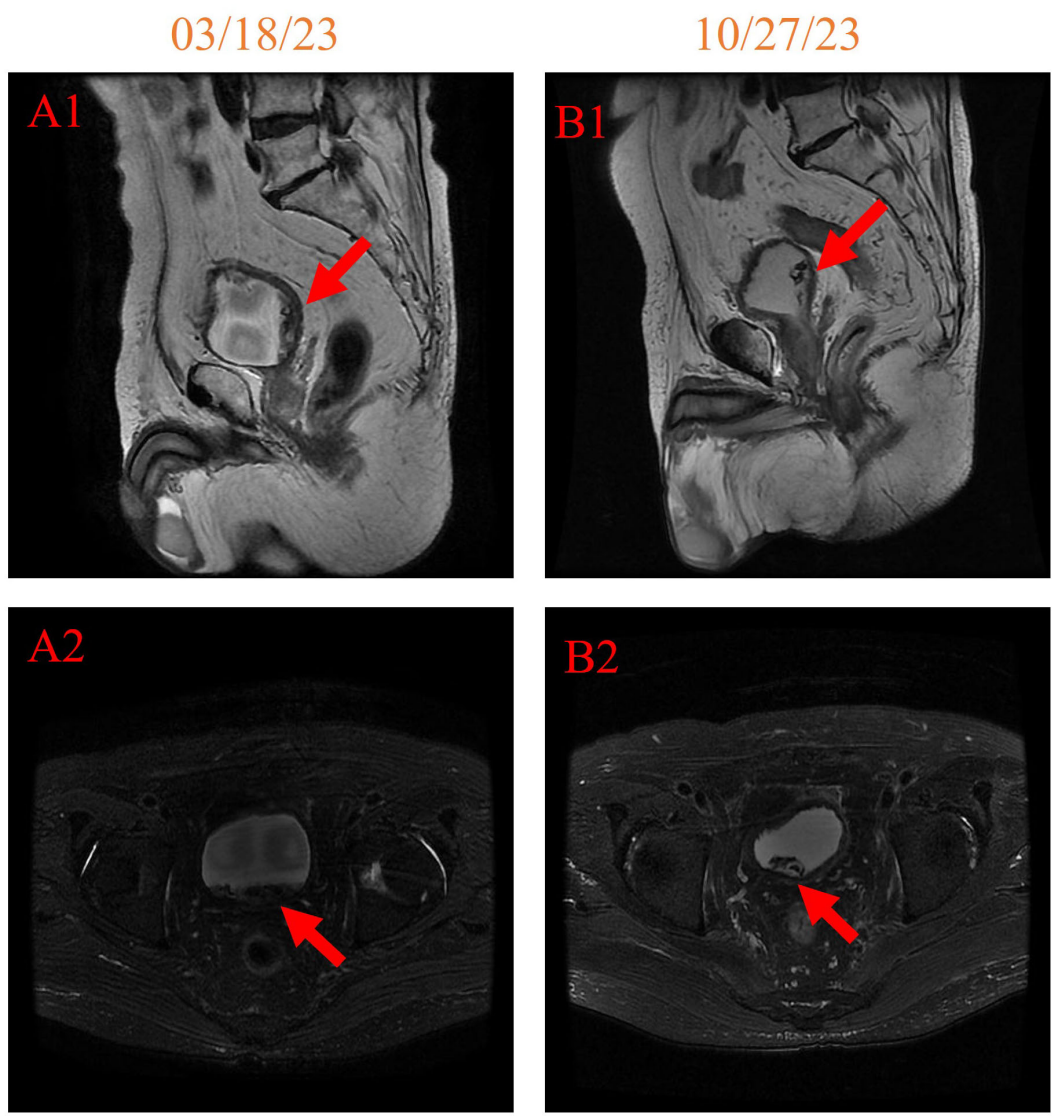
pelvic MRI imaging on 18 March 2023 [(A1, A2) 158 days after initiating HBOT] and 27 October 2023 [(B1, B2) 381 days after initiating HBOT].
